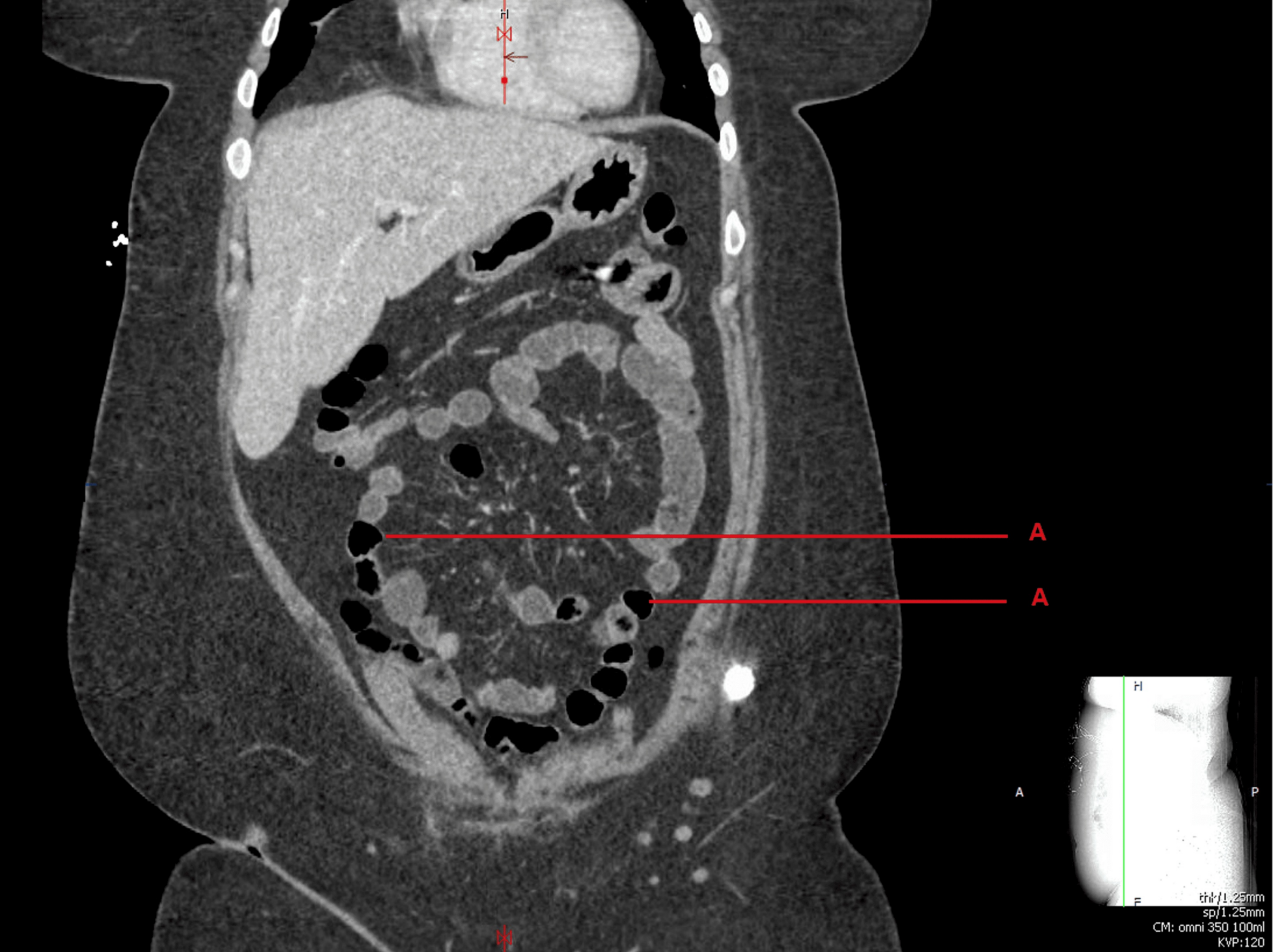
CT abdomen/pelvis on day 1 of admission showing bowel loops with air-fluid levels and colonic fluid accumulation (indicated by the red line marking A).
